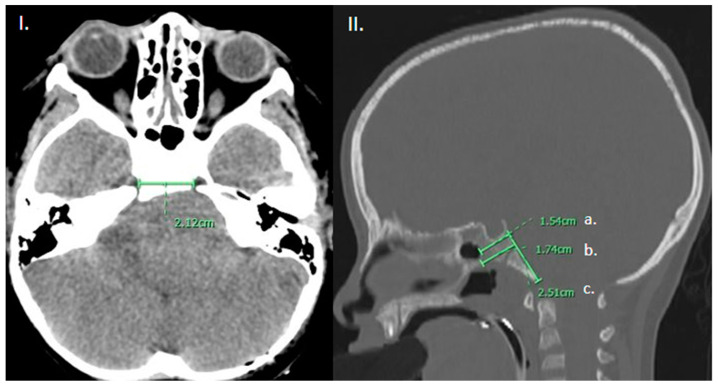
(I). Axial CT brain demonstrating measurement of the intercarotid distance. (II). Sagittal CT head, bone window in the mid-sagittal plane demonstrating measurement of the (a) thickness of the clivus at the level of the sellar floor as well as (b) at the thickest portion measurable and (c) height of the clivus from sellar floor to basion.
